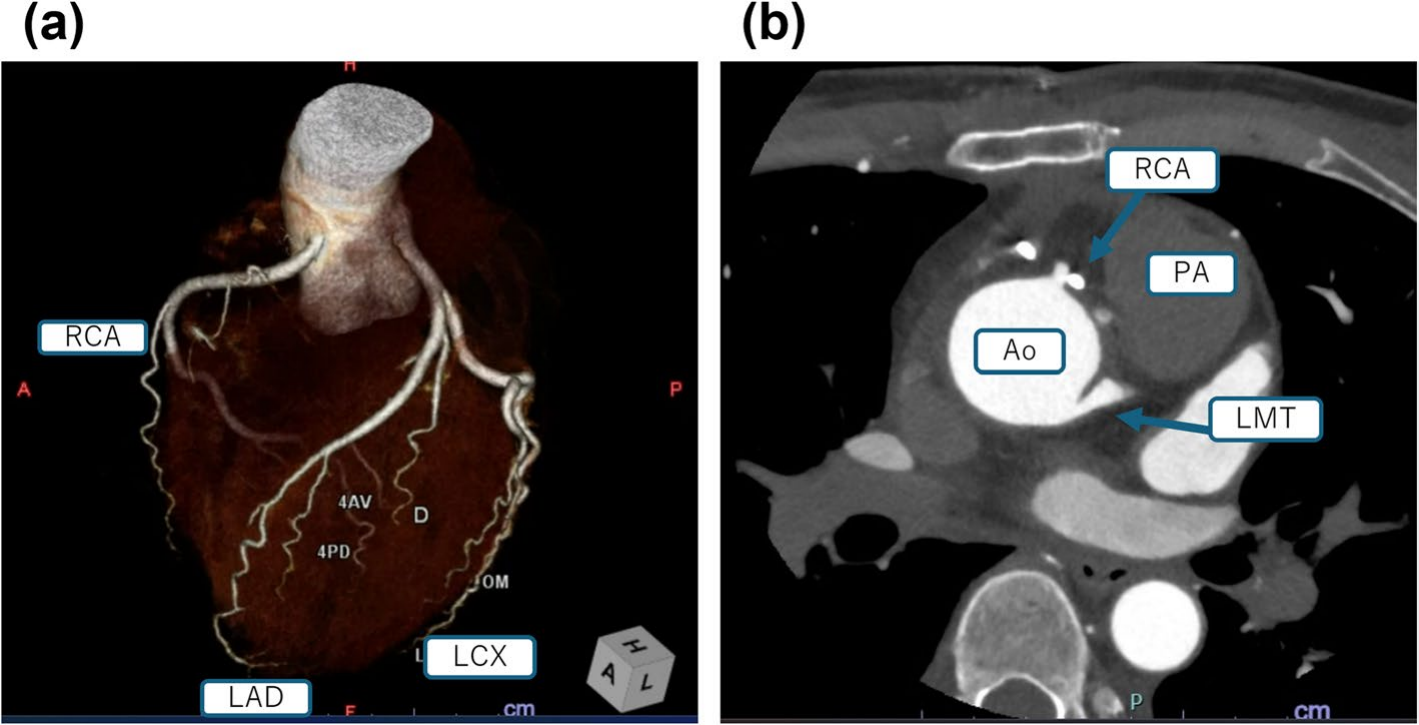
Postoperative coronary CTA findings. a, b The coronary artery was reimplanted in its normal position. Imaging showed good patency. RCA, right coronary artery; LAD, left anterior descending artery; LCX, left circumflex artery; LMT, left main trunk; Ao, aorta; PA, pulmonary artery
